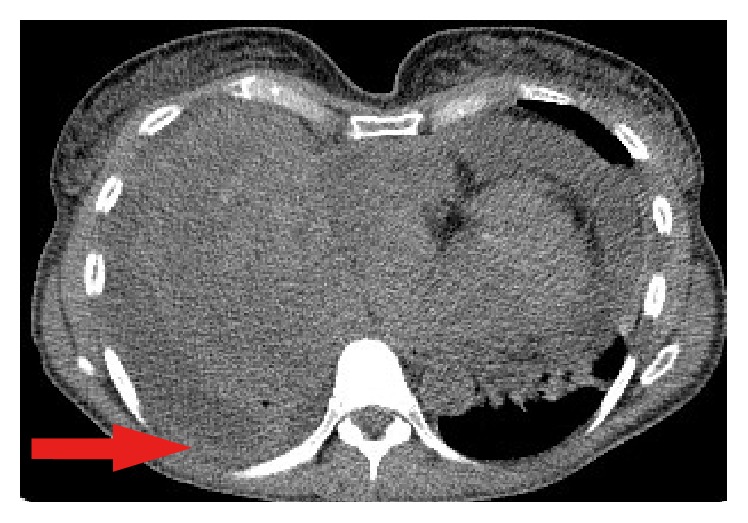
Axial CT demonstrating bilateral pulmonary effusions secondary to sarcoma.
